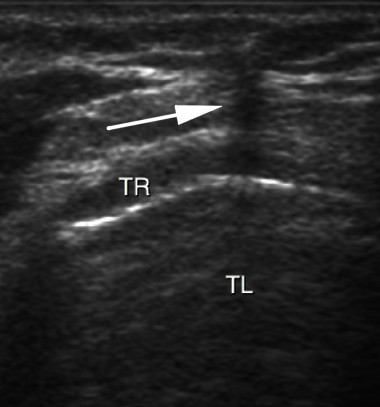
Real-time ultrasound guidance using an out-of-plane approach. Progression of the needle is determined by a distinct acoustic shadow (arrow). TL, trachea lumen; TR, tracheal ring.
